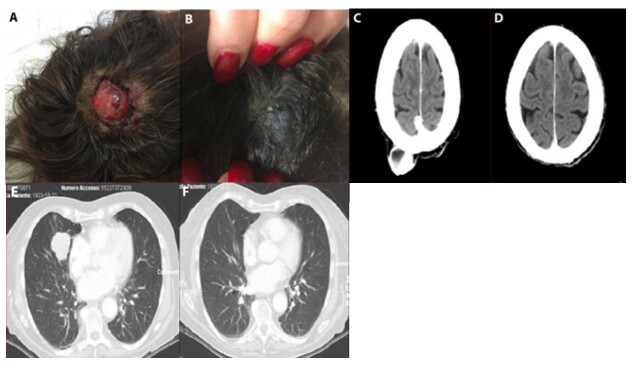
(A, B) Development of response in a representative patient with BRAF mutant metastatic melanoma with lung and skin metastases: response achieved as clinically evident at the 7th week from the beginning of treatment. (C–F) CT scan performed 3 months after the beginning showing the complete clearance of lung metastases
